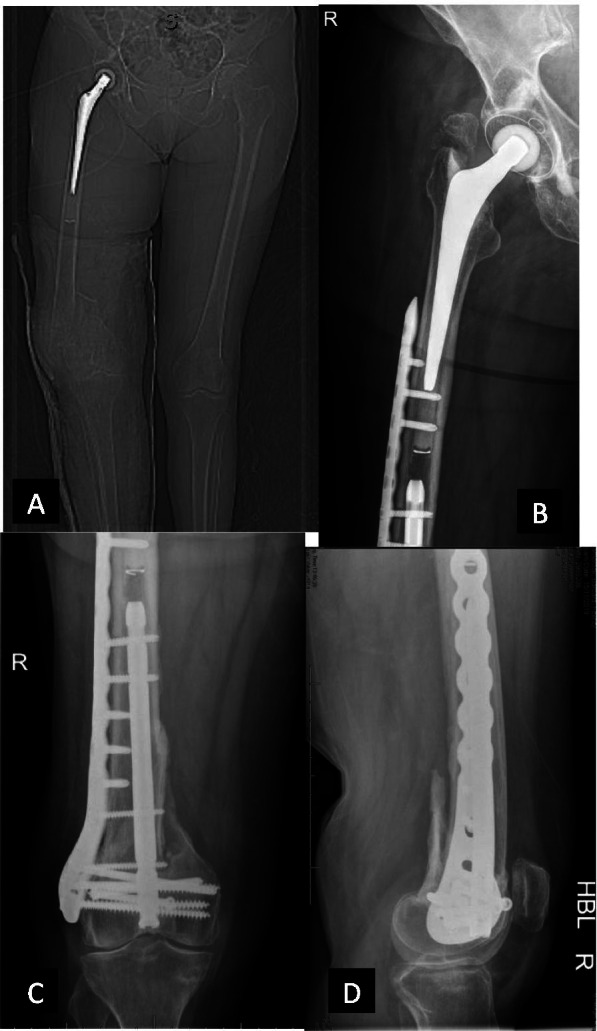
A periprosthetic femoral fracture (Vancouver type C). A Preoperative X-ray. B The fracture is fixed with an intramedullary nail. C Distal femur on anteroposterior X-ray. D Latera view
